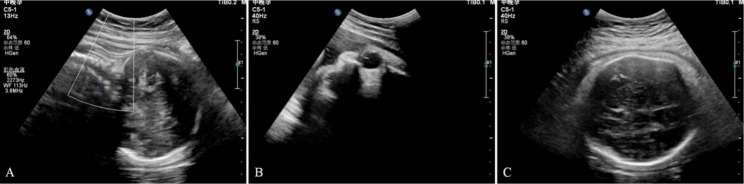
A Transabdominal ultrasound image of the foetal spine to determine the foetal head position. B Transabdominal ultrasound image of foetal orbits to determine foetal head position. C Transabdominal ultrasound image of foetal intracranial structures to determine foetal head position
